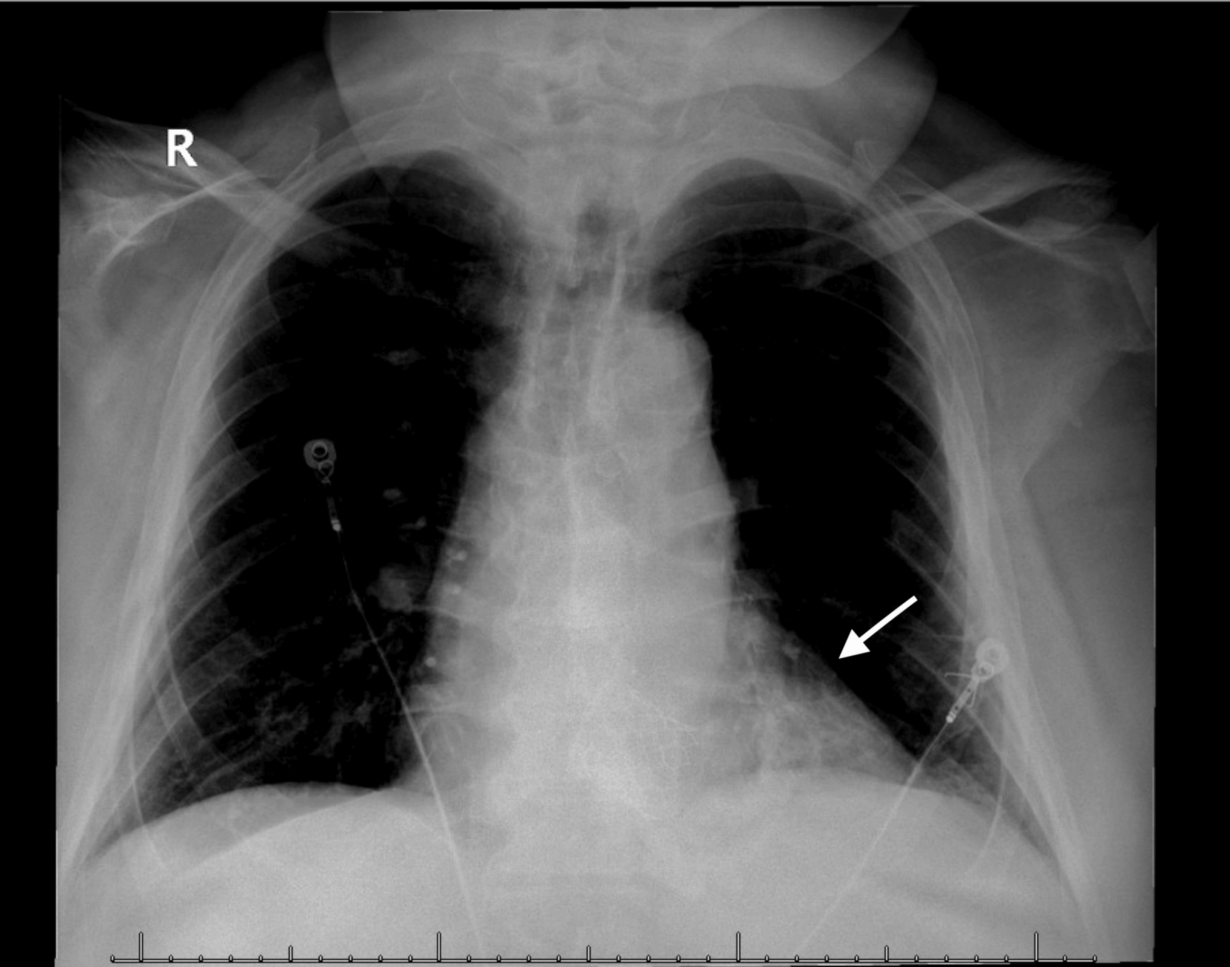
A/P chest X-ray read minimal left basilar atelectasis (arrow)
